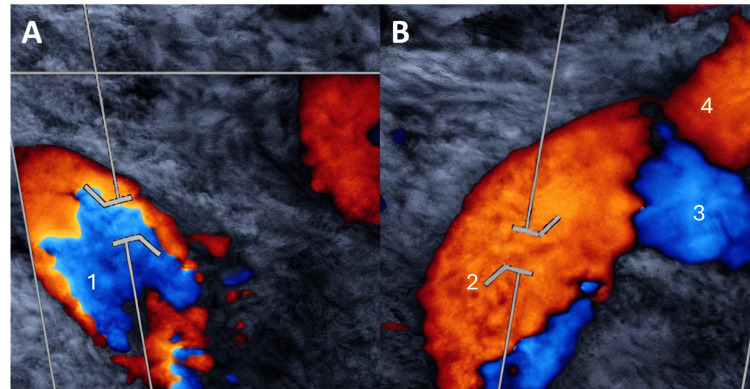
Carotid duplex ultrasound. (A) Color Doppler ultrasound image of the right supraclavicular window demonstrating the right subclavian artery (1). (B) Color Doppler ultrasound image showing the division of the left brachiocephalic trunk (2) into the left subclavian artery (3) and the left common carotid artery (4).
